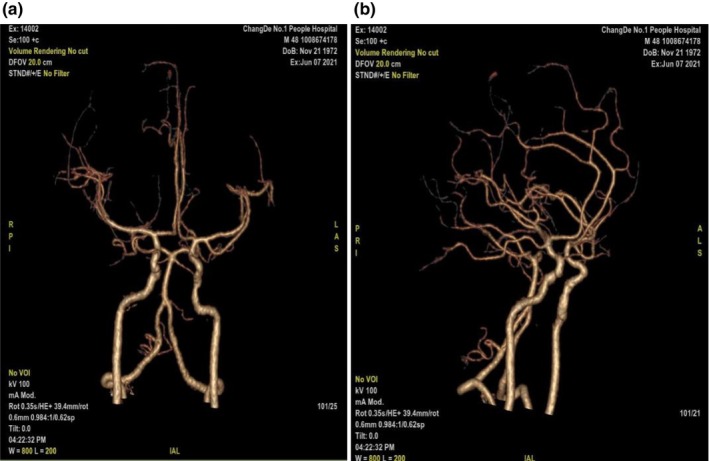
Cerebral CT angiography reveals: No obvious intracranial aneurysm or vascular malformation was observed.
